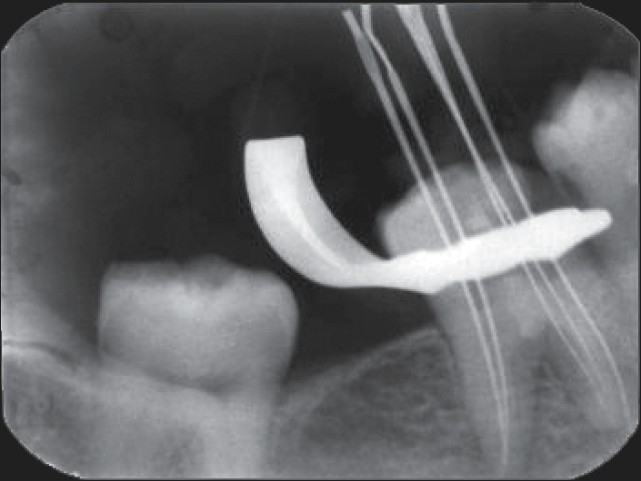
Working length radiograph taken with tube shift (distal shift) technique
